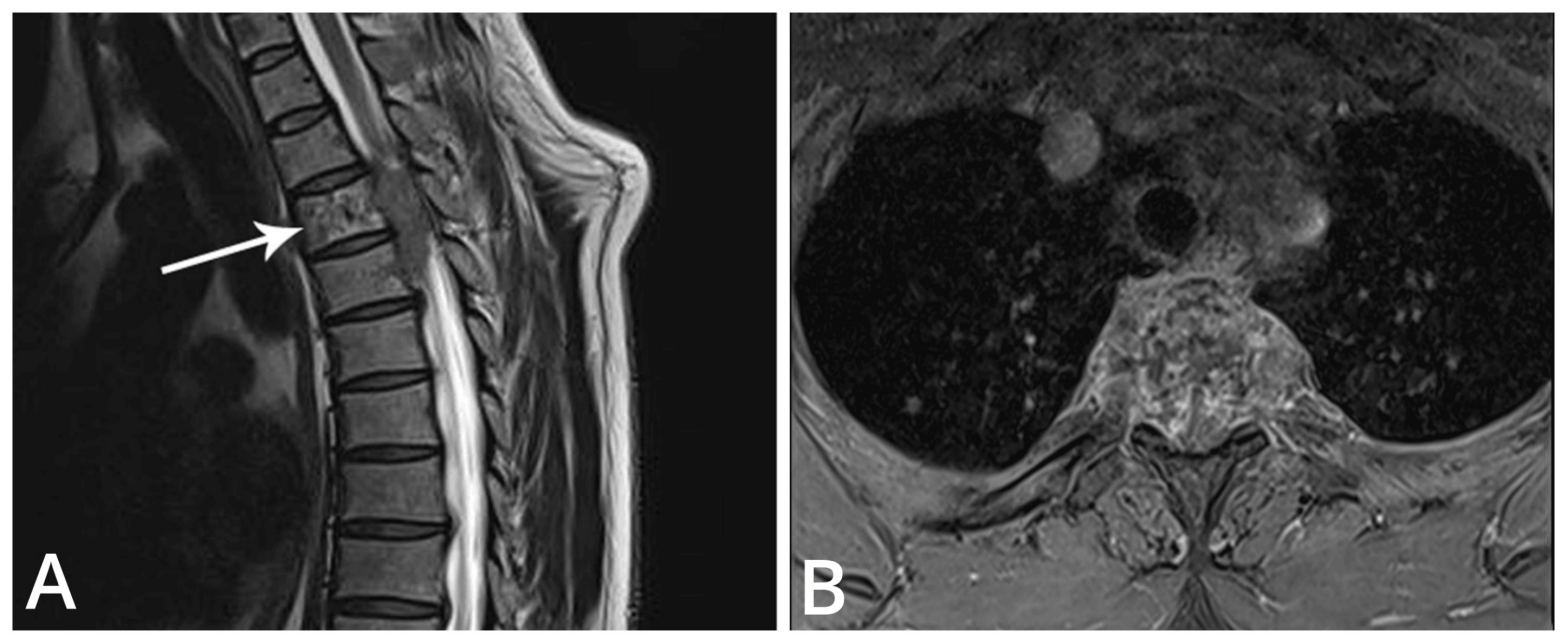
MRI of the patient showing metastatic involvement of the third thoracic vertebra. (A) Sagittal plane; (B) transverse plane. MRI: magnetic resonance imaging
